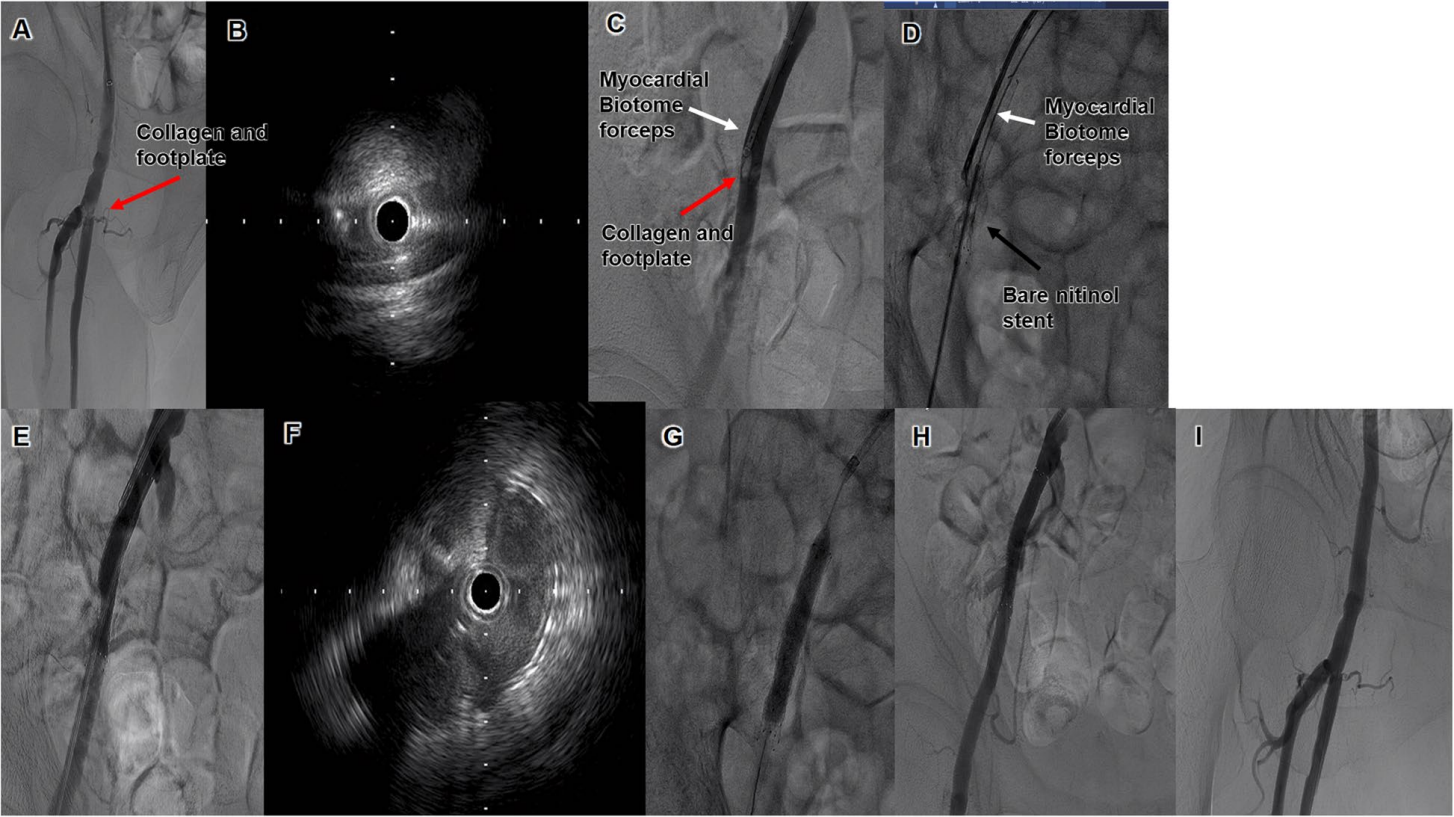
Angiogram of Case 2 after the Angio-Seal drop. A The Angio-Seal collagen has stopped at the bifurcation of the deep femoral artery (DFA). B Intravascular ultrasound (IVUS) observation from the superficial femoral artery (SFA) shows that the Angio-Seal collagen occupies the SFA and almost excludes the SFA. C A biotome grasps the Angio-Seal collagen and pulls it up to the external iliac artery (EIA). D. While the biotome forceps grasp the collagen, a 10.0- × 60-mm stent (SMART^®^; Cordis, USA) is deployed to the EIA and the Angio-Seal collagen is positioned between the stent and the vessel wall. E After stent deployment, the stent expansion is not completed. F IVUS observation of the portion of the stent where the collagen is inserted shows that the collagen is pushing the stent. G Post-dilation of the stent. H-I The final angiography shows good antegrade flow with no dissection or residual stenosis. The stent is well dilated and there is no remaining material at the bifurcation of the SFA and the DFA
